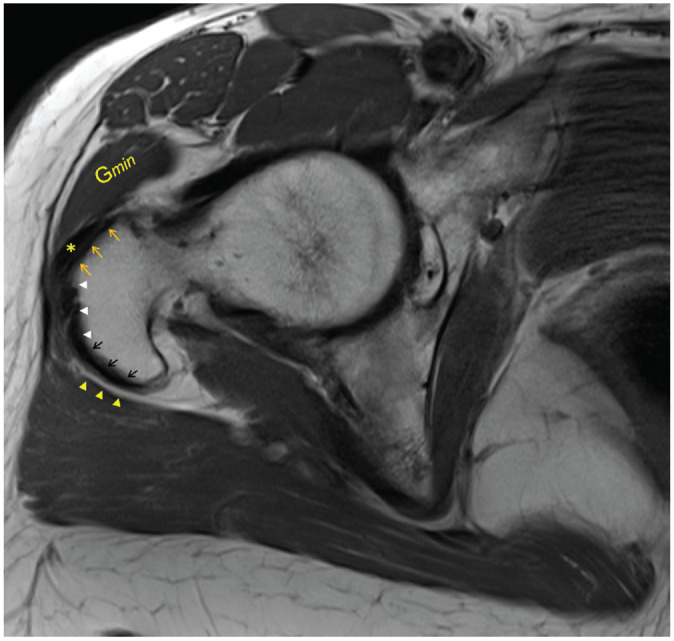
Axial T1-weighted magnetic resonance image of a right hip with intact hip
